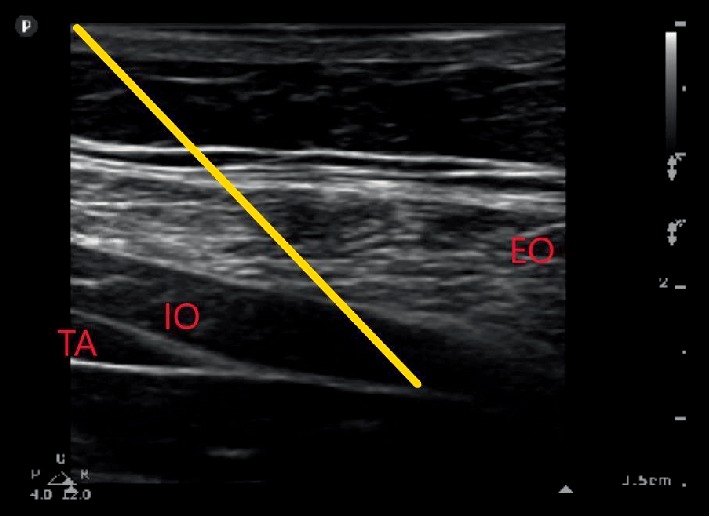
Ultrasound image obtained to place nerve catheters. EO = external oblique muscle. IO = internal oblique muscle. TA = transversus abdominis muscle. Yellow line depicts trajectory of needle into posterior aponeurosis of transversus abdominis muscle.
